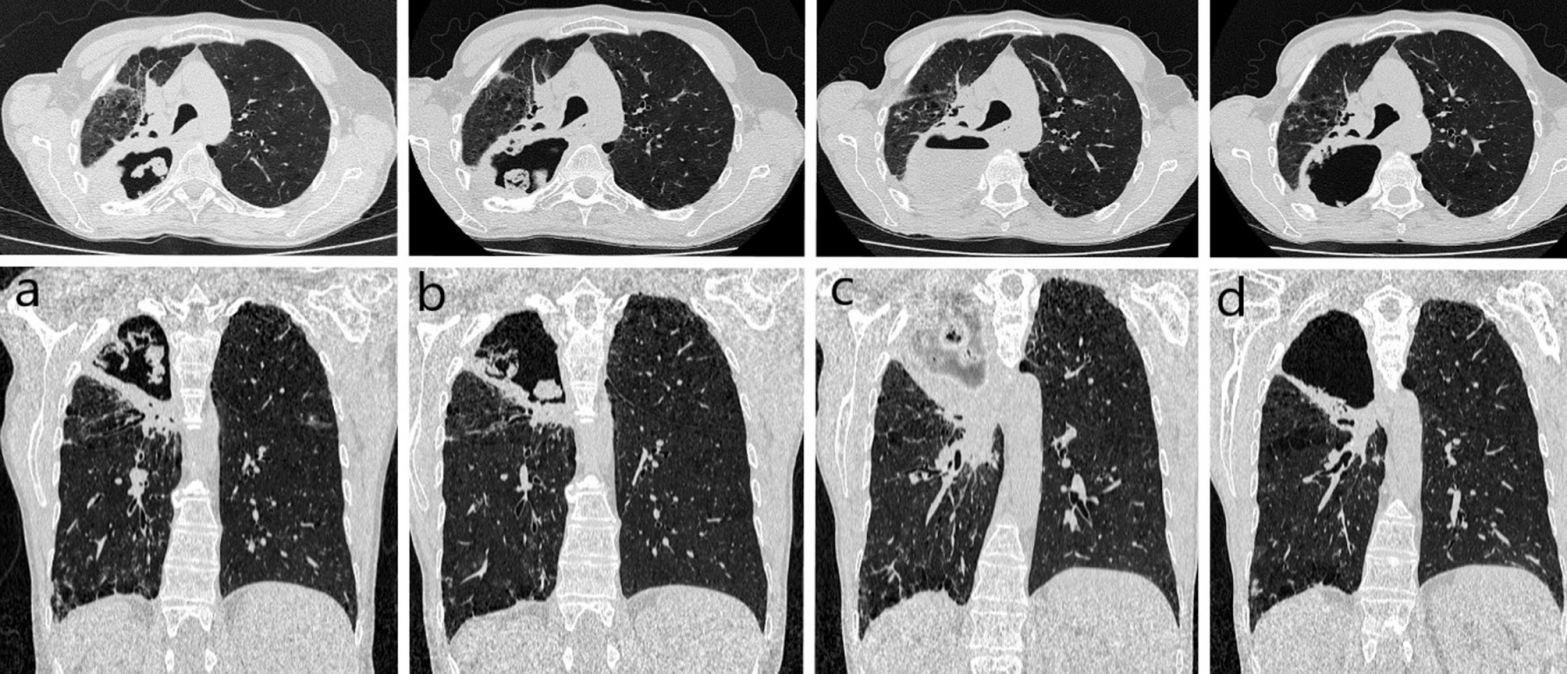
Controlled chest CT during second-line treatment in 2021: (a) at the beginning of the therapy – cavity with thick walls size 69 × 47 mm, concomitant mass on the posterior wall-size 22 × 9 mm, quantitative progression of the surrounding consolidations compared to the previous image; (b) after 3 months – cavity size 70 × 66 mm, the mass inside larger and more irregular (progression); (c) after 4 months – circumscribed opacity with an air-fluid level size 95 x 75 mm in the place of the previously described cavity (further progression, abscess); (d) after 5 months – cavity with thick wall much smaller, the mass inside size 28 × 20 mm, no fluid was visualized (regression).
